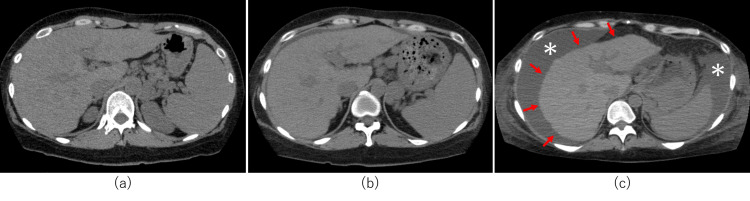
CT images of Case 1 (a) CT image on admission: coarse liver parenchyma, mild splenomegaly, gastro-renal shunt, and mild splenomegaly are seen. (b) CT image after four months: mild hepatic atrophy and slight ascites are present. (c) CT image after eight months: liver atrophy is rapidly progressing (red arrows) and severe ascites is seen (white *) CT: computed tomography
